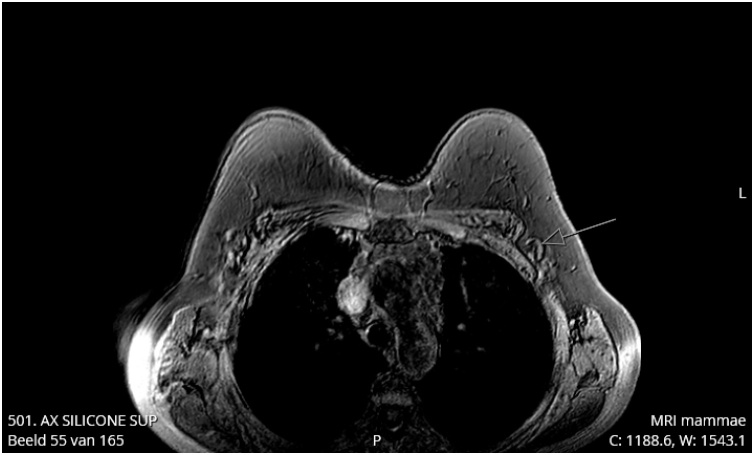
Follow-up breast MRI after three months. No evident identifiable pathologies such as macro level siliconomas as seen on earlier MRI. Possibly one lateral lymph node (left) with minimal silicone residuals.
